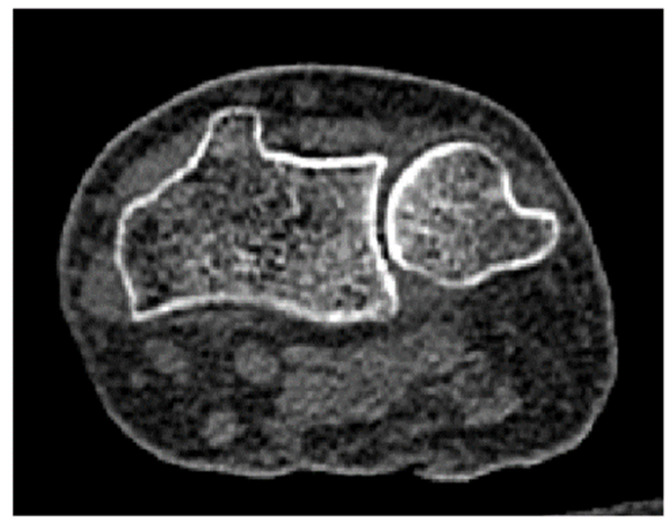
A CT image (512 × 512 pixels) of subject’s wrist showing the radius and ulna. Seventy slices of CT images were utilized in the present study.
